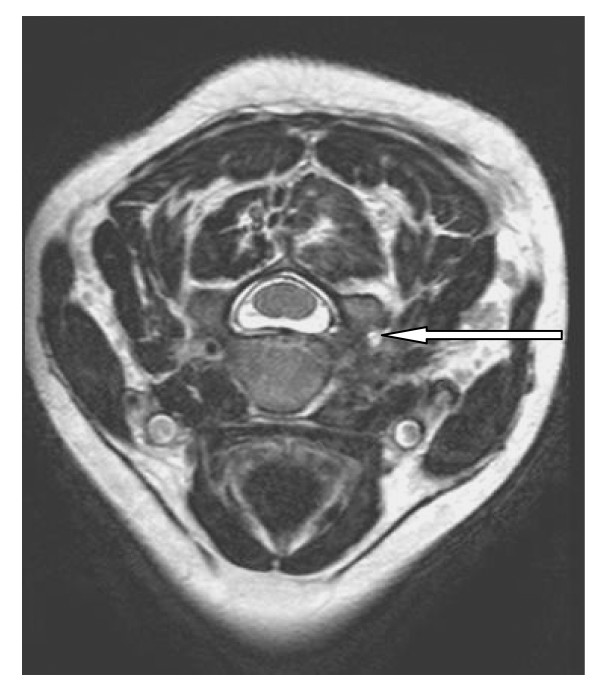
MRI of the cervical spine. T2-weighted image shows high signal intensity (white arrow) of the right vertebral artery. There is an intramural thrombus plus occlusion of the lumen (Grade four dissection). There is no intraluminal thrombosis.
